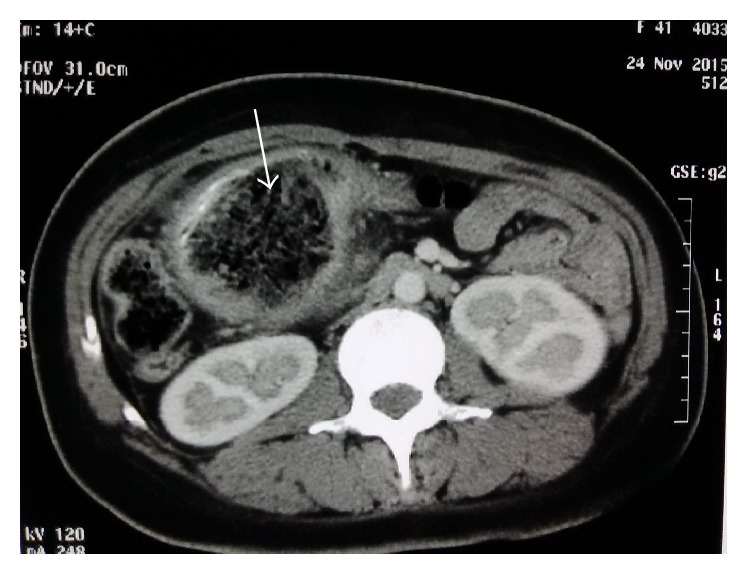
Injected abdomen CT scan showing limited spongiform mass with calcifications (textiloma) in the colon lumen.
